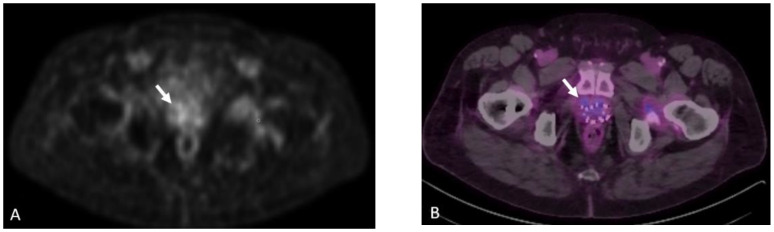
Sixty-four-year-old male with history of prostate cancer, status post-brachytherapy, presenting with rising PSA level. 18F DCFPyL PET (A) and fused PET/CT (B) images show multifocal radiotracer uptake in the mid-prostate gland (arrows). This uptake subsequently resolved on the follow-up scan (not shown). It was later revealed that the patient had undergone a transrectal prostate biopsy three weeks prior to our study, confirming the uptake to be secondary to inflammation from the recent biopsy.
